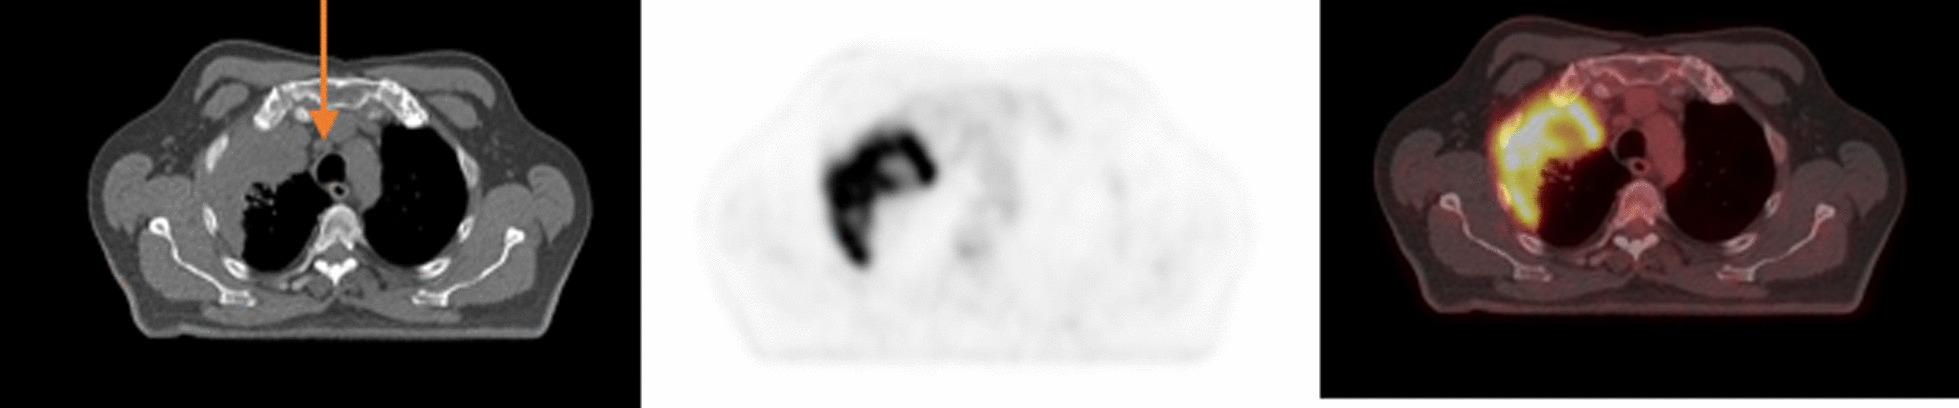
True negative FDG-PET/CT and true negative EBUS/TBNA. A case of poorly differetiated lung adenocarcinoma. Axial CT scan showed prominent right upper paratracheal lymphnode (arrow). Axial FDG-PET and PFDG-PET/CT didn’t show concerning hypermetabolic features (SUVmax: 1.2) in this lymph node. EBUS/TBNA turned negative for metastases in this lymph node that was confirmed during lymph node dissection
